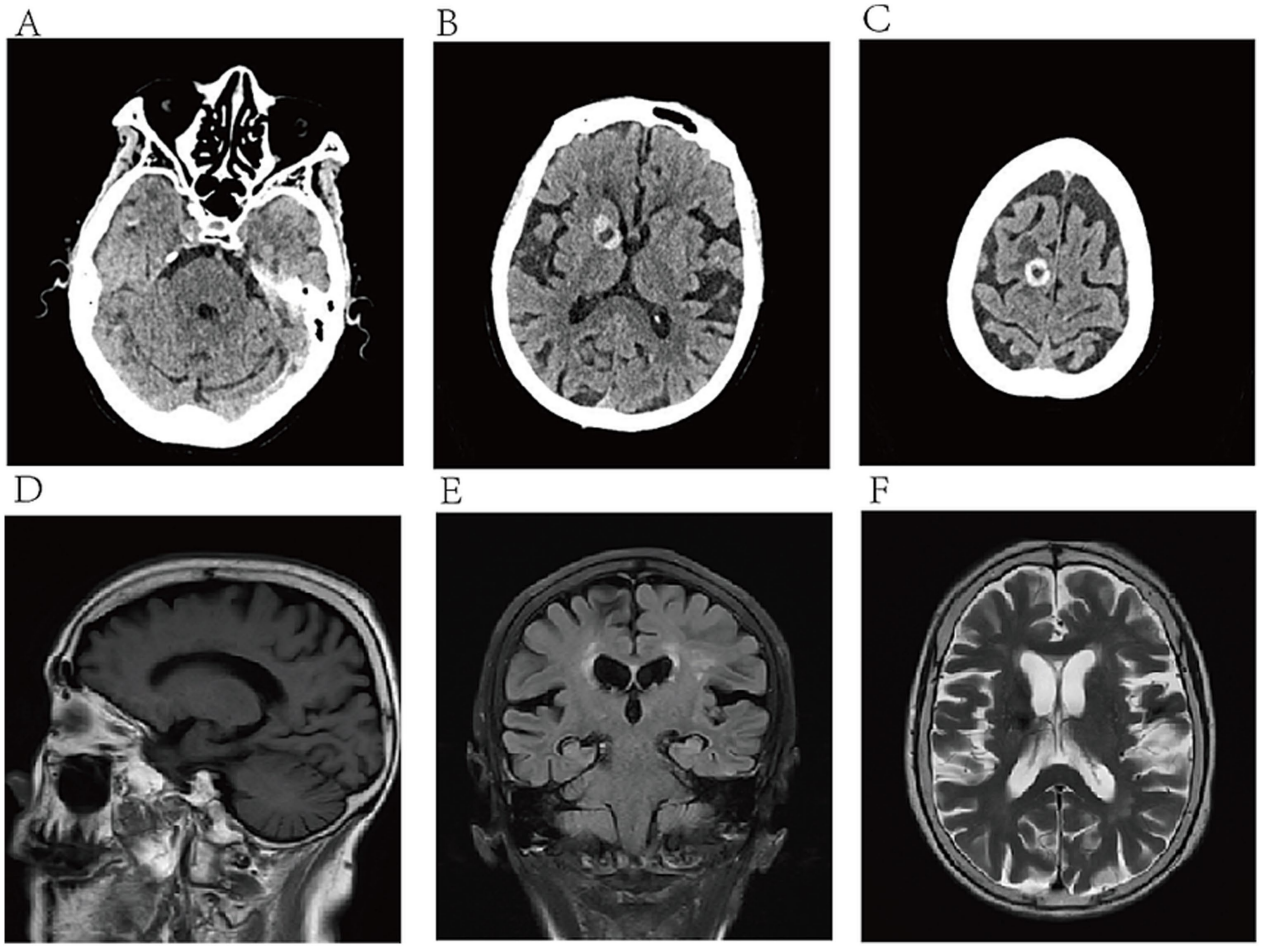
(A–C) CT scans obtained on March 30 revealed multiple intracranial nodular lesions; (D–F) MRI scans acquired on October 6 showed that most intracranial lesions had decreased in size compared with prior images, with some completely resolved.
